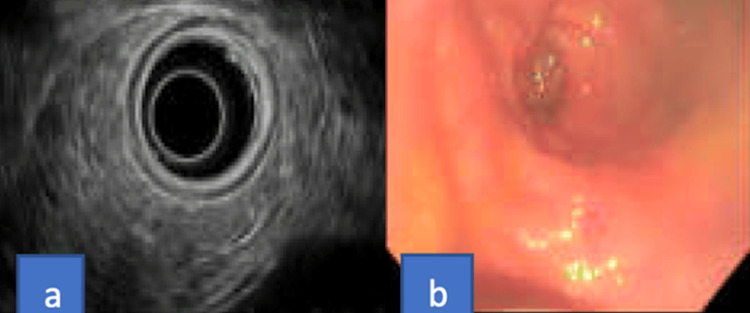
(a) Endosonographic image and (b) endoscopic image showing a normal rectum and recto-sigmoid with no evidence of submucosal mass.
